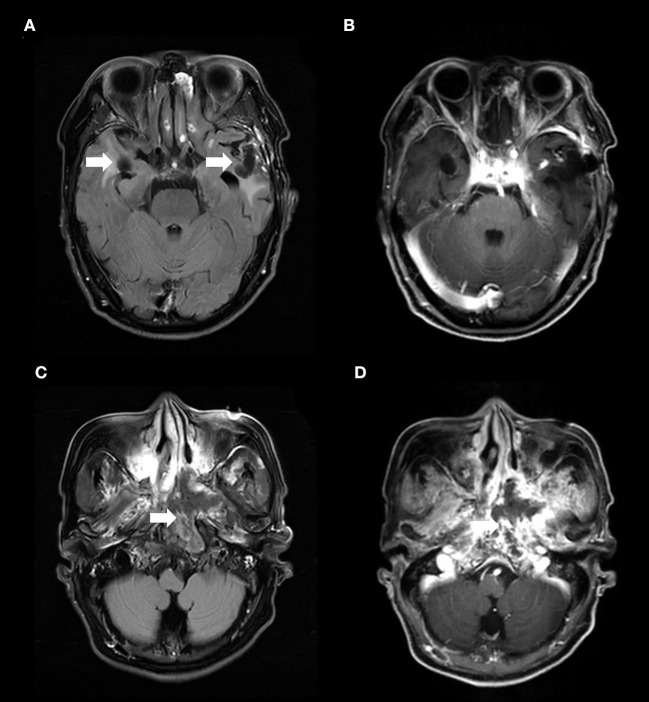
(A) The axial T2-weighted FLAIR imaging demonstrated irregular hypointense signal changes with patchy edema around them in the bilateral temporal lobes (arrows) and (B) without contrast enhancement in the similar brain structures. The MRI abnormalities on bilateral temporal lobes had been present for approximately 5 months before the patient's disturbance of consciousness. These abnormalities were presentation of radiation-induced brain necrosis and were not associated with *E. miricola* encephalitis. (C) Nasopharynx T2-weighted FLAIR imaging showed a heterogeneous signal mass in the left nasopharyngeal lateral wall (arrow). (D) T1-weighted nasopharynx MRI with contrast enhancement showed enhancing lesions in the mass (arrow). FLAIR, fluid-attenuated inversion-recovery.
